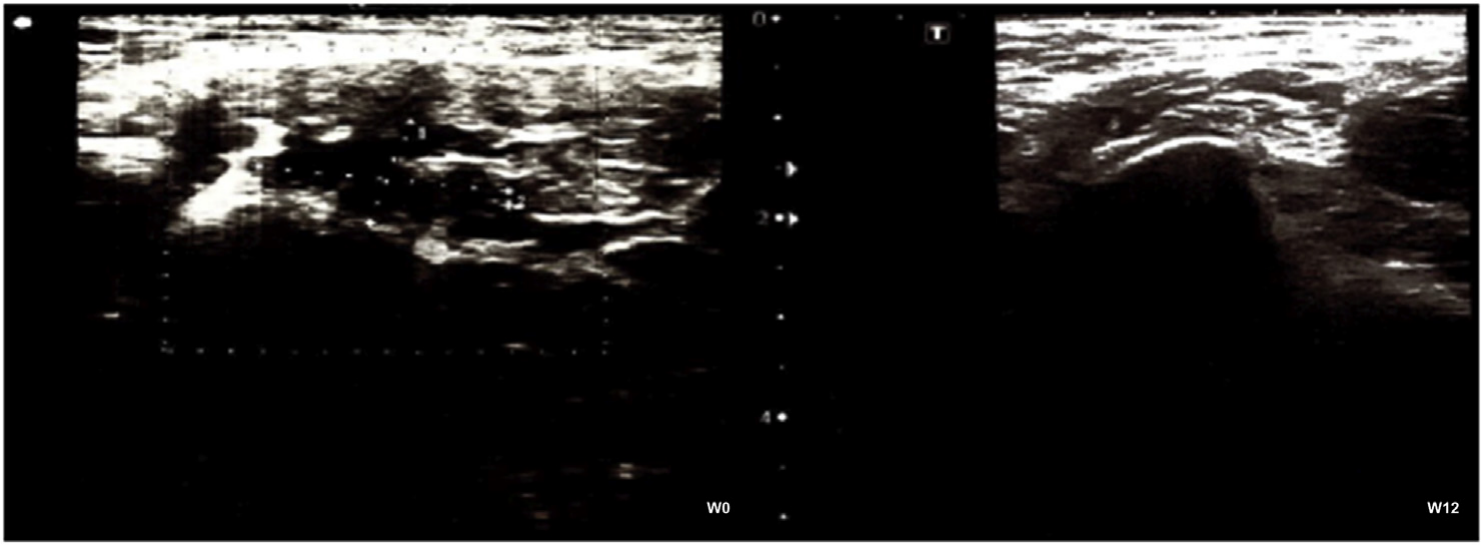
Ultrasound examination. Representative ultrasound examination at week (W)0 and at W12 of a professional roller hockey player who presented calcifications and liquid deposit before beginning the treatment. At the end of the treatment, calcifications and liquid deposit were substantially reduced.
